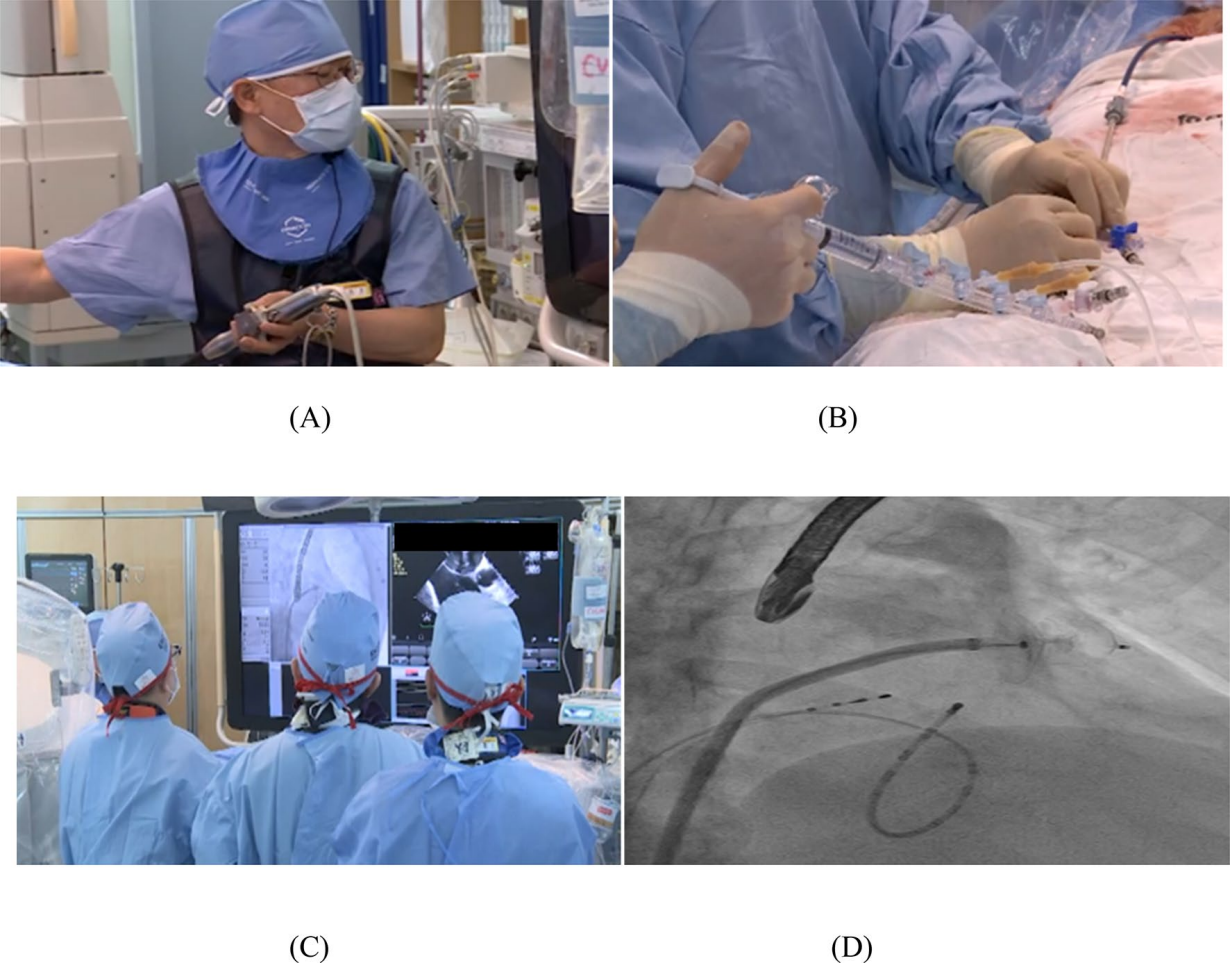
LAAO was performed using a device of the predicted size. (A) A cardiologist performing TEE during the procedure. (B) Another cardiologist injecting saline, medicine, and medium contrast through the manifold. (C) Checking of fluoroscopy and TEE in real time during the procedure. (D) Confirmation of the device insertion predicted by fluoroscopy correctly into the LAA. *LAA* left atrial appendage, *LAAO* left atrial appendage occlusion, *TEE* transesophageal echocardiography.
